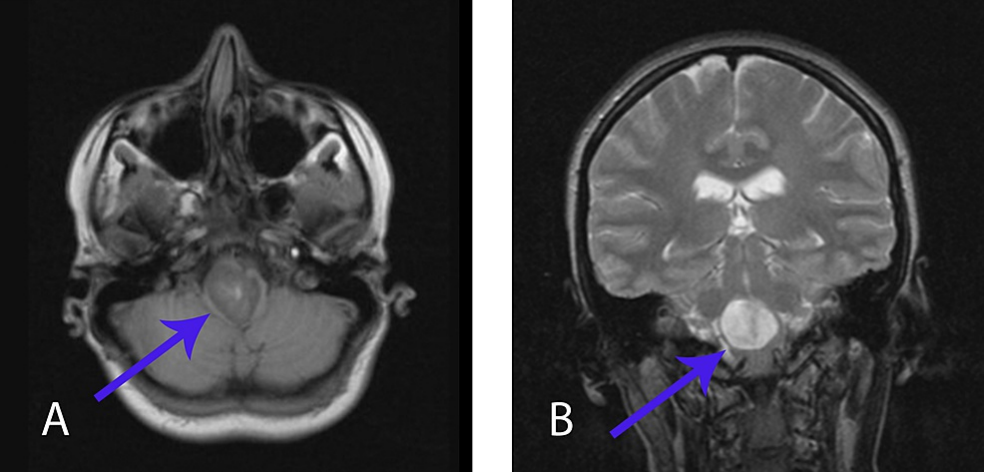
MRI, two months after the first surgery A – MRI Т1 axial view; B – MRI Т2 frontal view
